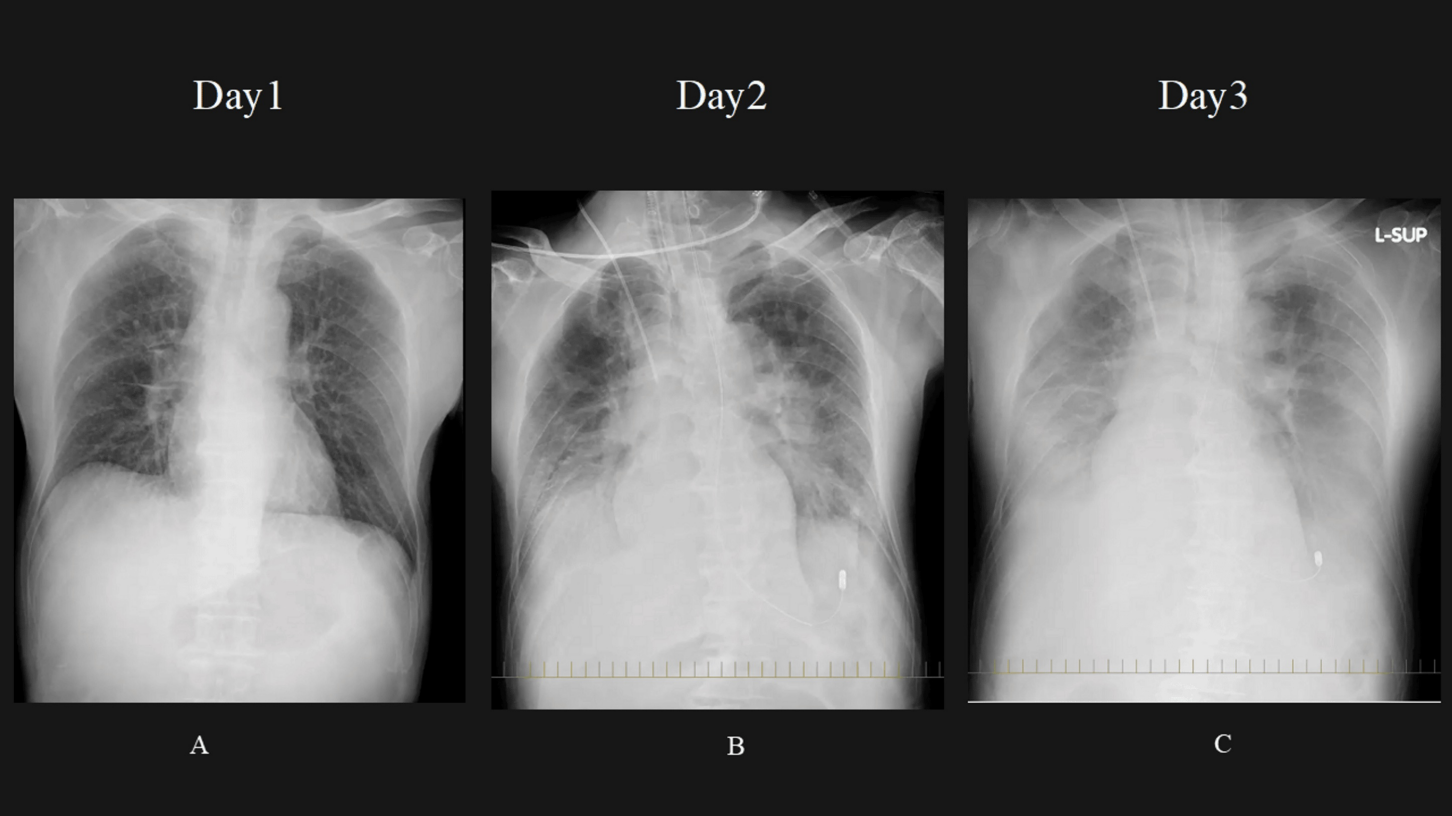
Changes in chest X-ray since admission Day 1 (A): The initial chest X-ray shows the baseline condition of the lung fields. Day 2 (B): Gradual worsening of lung field infiltration shadow is observed. This is partly attributed to volume loading, which may have caused an increase in the density or the extent of the shadow in the lungs. Day 3 (C): Further progression of lung field infiltration is noted, with continued worsening from the previous days. The volume loading effect may still be contributing to this change.
